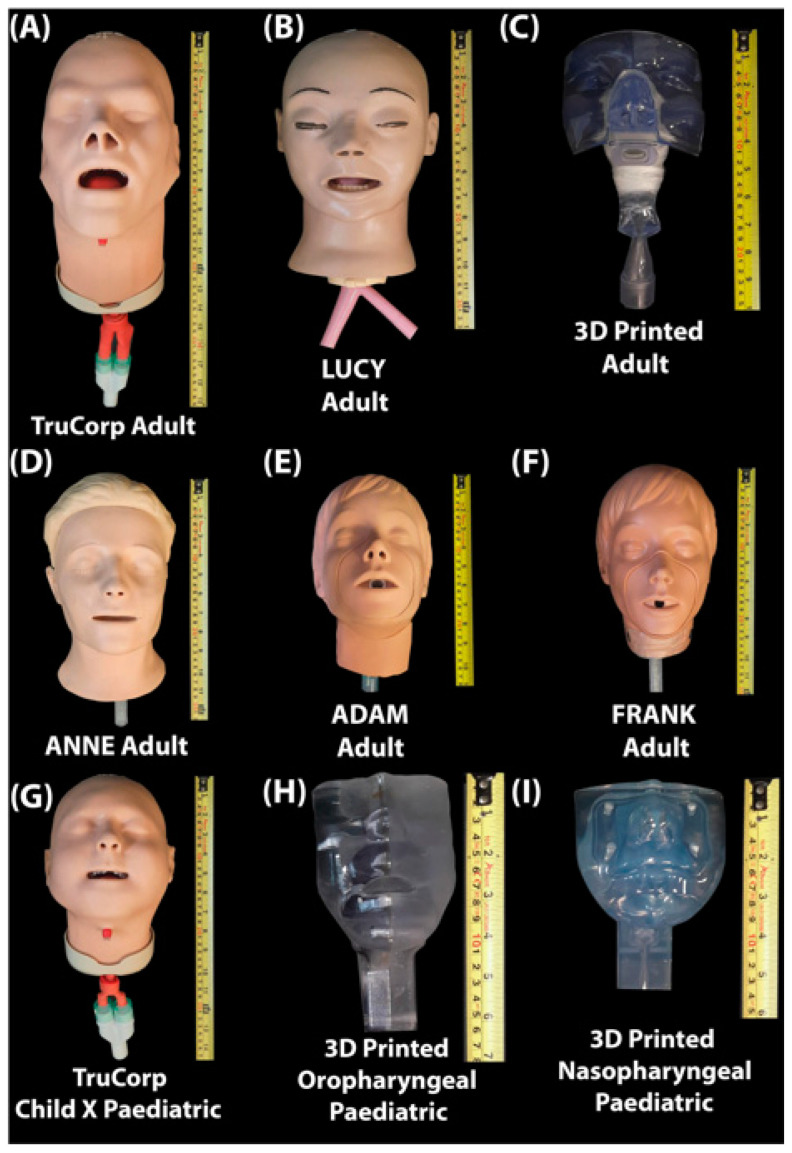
(A) TruCorp AirSim Advance X (AA91100X, TruCorp^®^, Craigavon, Ireland); (B) LUCY (Life/form^®^ Adult Airway Management Trainer, Head Only, SKU: LF03603, Nasco Healthcare, Saugerties, NY, USA); (C) 3D Printed anatomically correct head model generated from an individual CT scan; (D) ANNE (Resusci Anne^®^ QCPR, Laerdal medical, Stavanger, Norway); (E) FRANK (Model HSM-A 1550, Michigan Instruments, Kentwood, MI, USA); (F) ADAM (Adult #2000, Simulaids, INC, Saugerties, NY, USA); (G) TruCorp AirSim Child X (AC 10006X, TruCorp^®^, Craigavon, Ireland); (H) 3D Printed anatomically correct oropharyngeal (Male 5 years) generated from an individual CT scan; (I) 3D printed anatomically correct nasopharyngeal (1688) head model generated from an individual CT scan.
